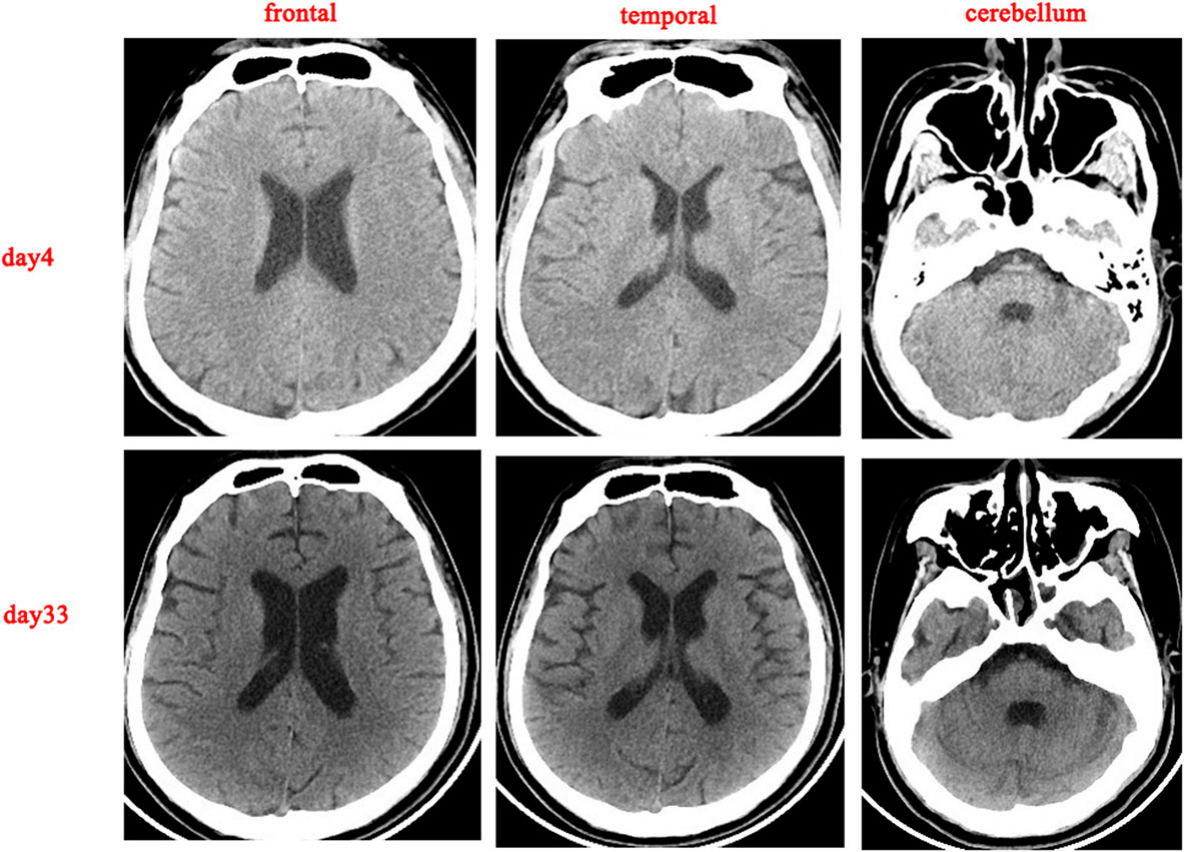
Computed tomography images of the brain showing atrophy of the temporal and frontal lobe and the cerebellum, comparing the computed tomography scan on day 4 to the computed tomography scan on day 33
